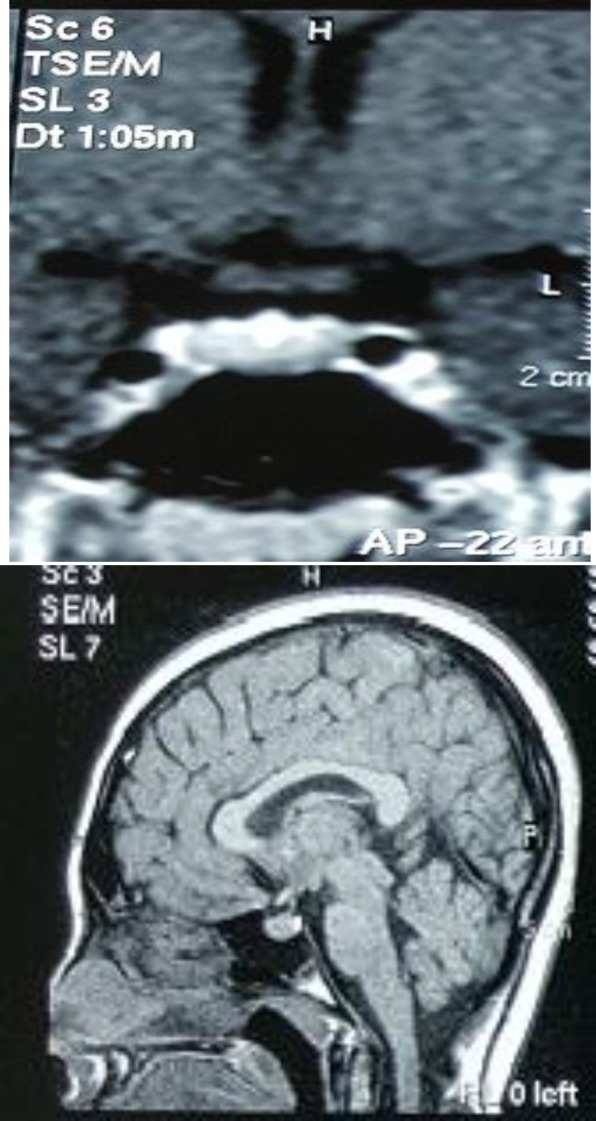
MRI (4 months after treatment) showed a small (2 mm) lesion at left pituitary lobe
